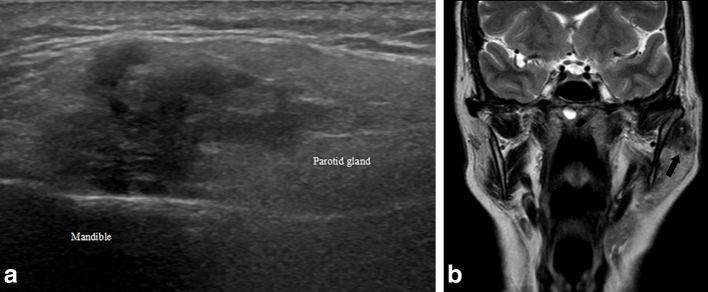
(a) Ultrasound image (longitudinal) demonstrating a poorly defined, irregular, hypoechoic lesion that corresponds to the primary parotid tumour, within the superficial parotid gland extending towards the deeper lobe. (b) Coronal *T*
